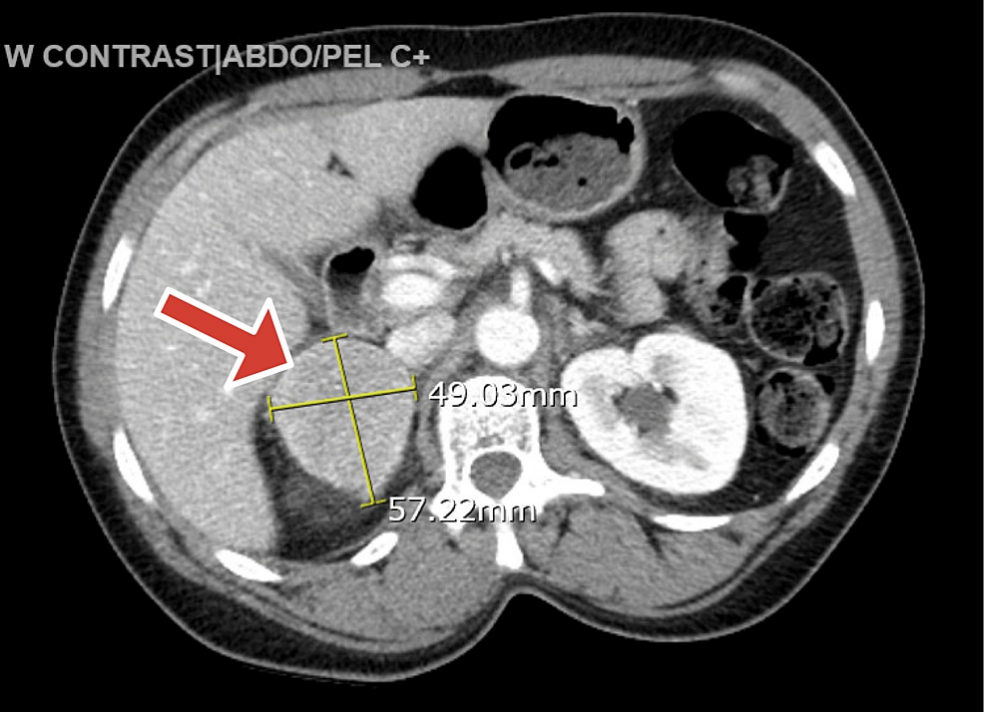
Computed tomography of the abdomen depicting the right-sided adrenal tumor
